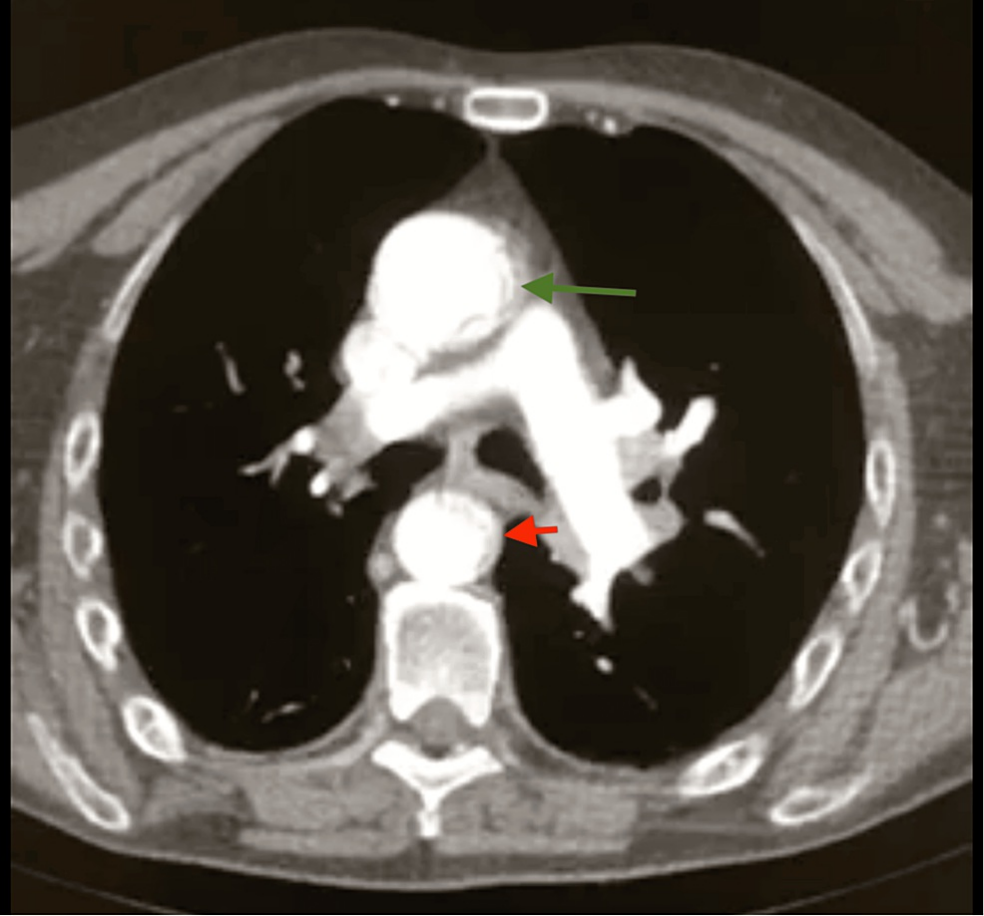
Thorax CT angiography demonstrating aortic dissection of the ascending aorta (green arrow) and descending aorta (red arrow), showing true and false lumen opacified with contrast
